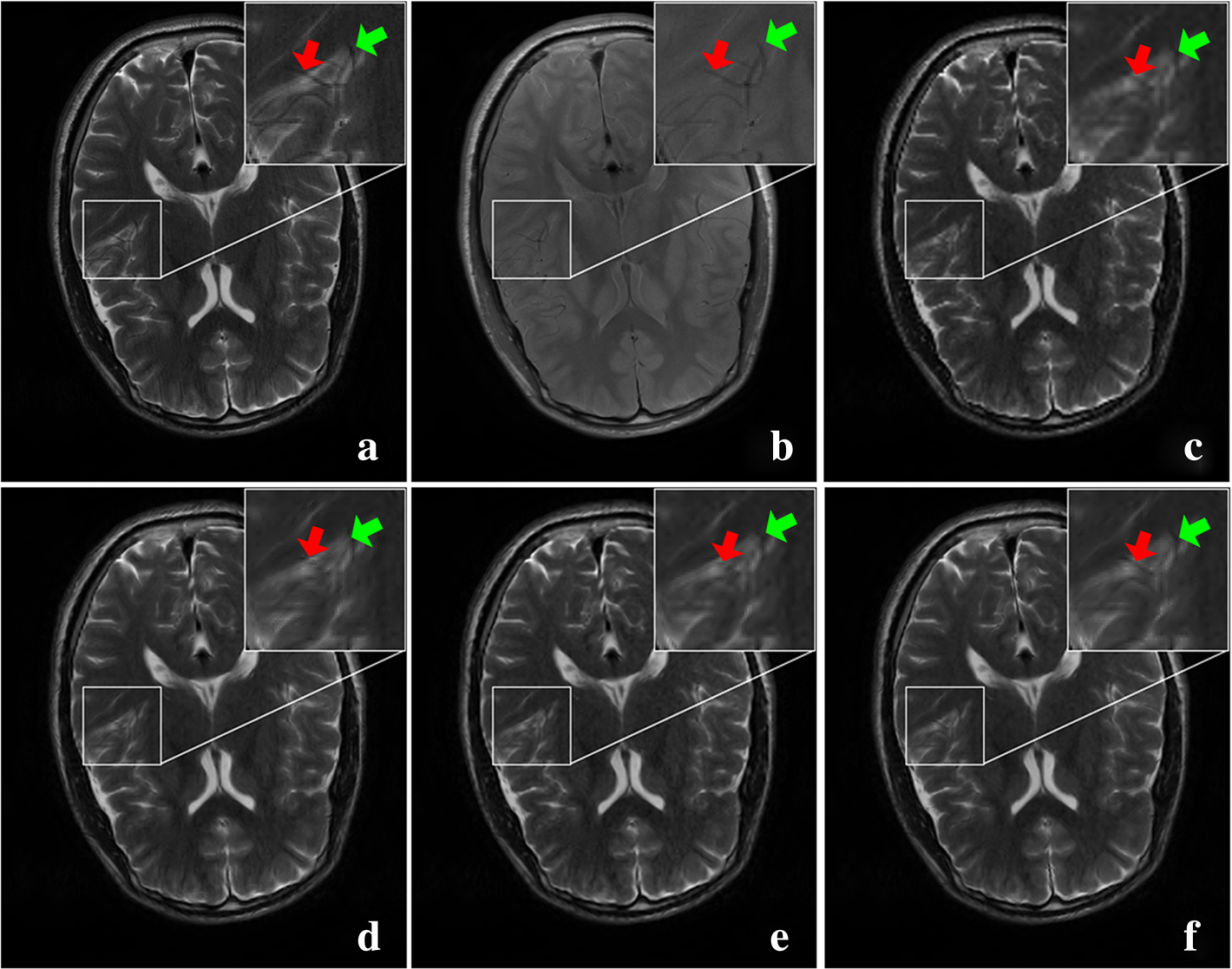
Another pair of T1 and T2 MRI images acquired on 3 T Siemens scanner. a HR of T2 image; b HR of T1 image; c-f are super-resolved images using the bicubic, NEDI, CGI, and the proposed method, respectively
